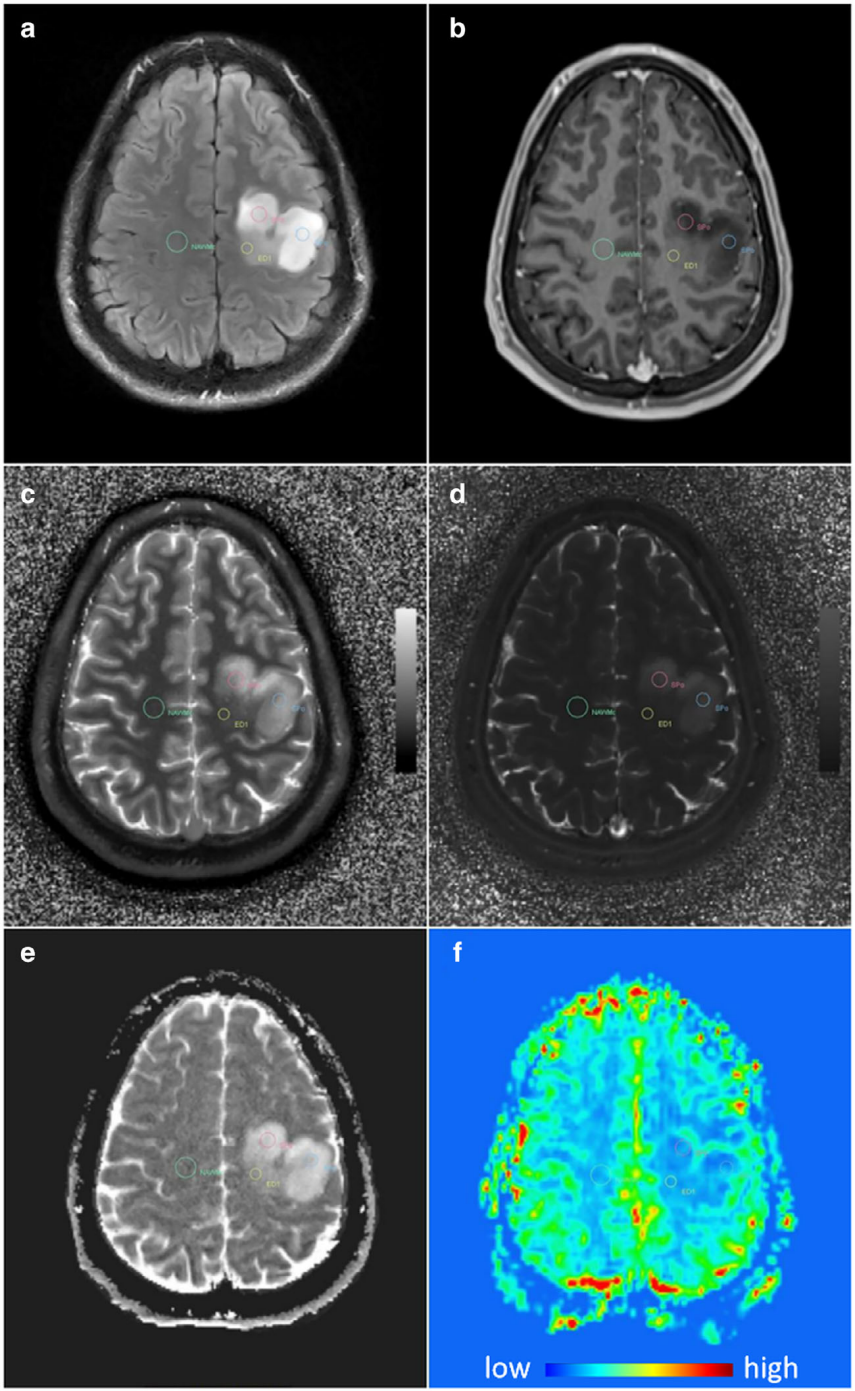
Quantitative maps derived from MRF in an astrocytoma, IDH‐mutant, show increased T1 and T2 values within the tumor mass. The MRF T1 map (c) and T2 map (d) are compared to FLAIR (a), T1w contrast‐enhanced MRI (b), an ADC map (e), and perfusion‐weighted imaging (f). Reproduced from Springer et al 2022 under CC license.^112^
